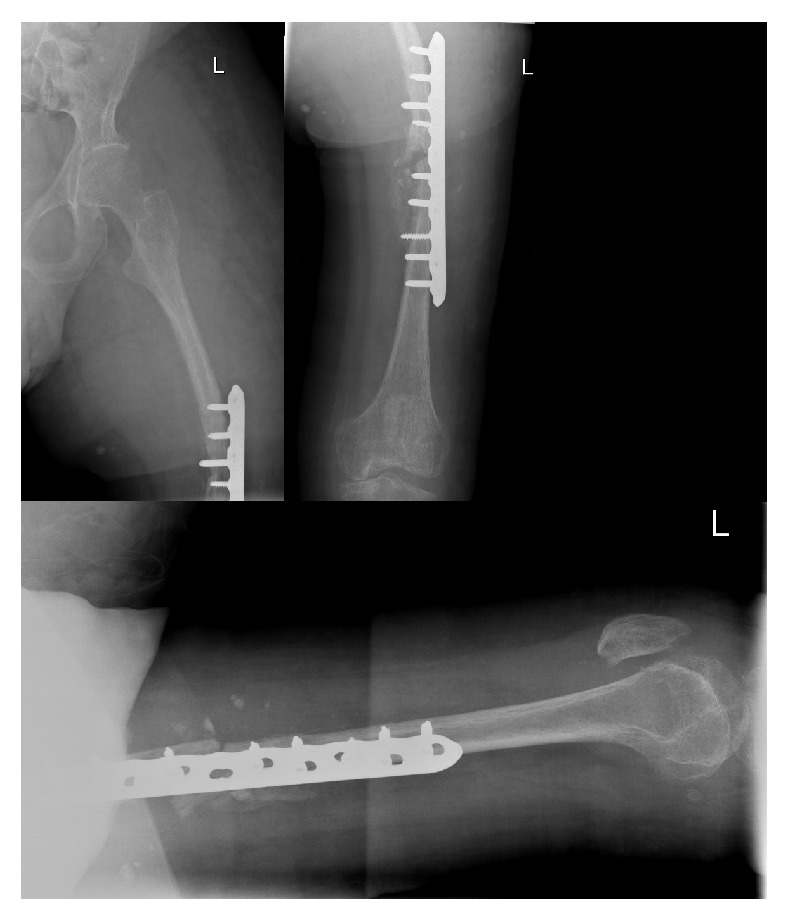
AP and lateral radiograph of the left femur immediately after open reduction, internal fixation.
